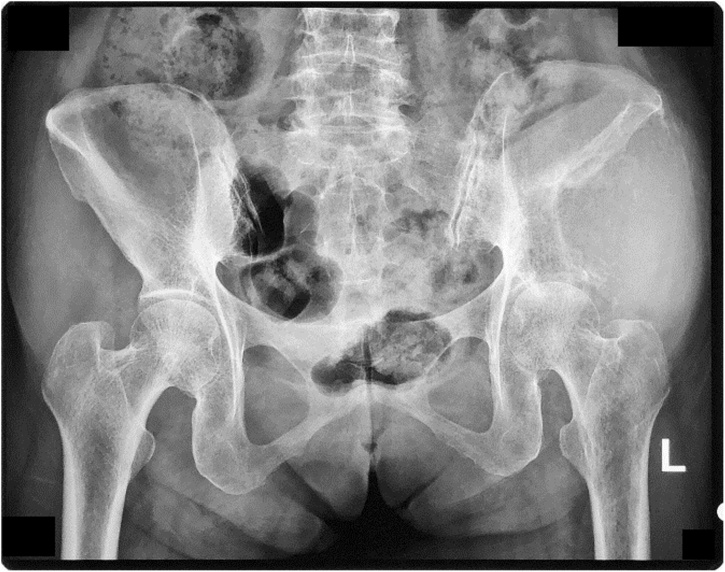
Pelvic Radiography Showing Destructive Mass.
